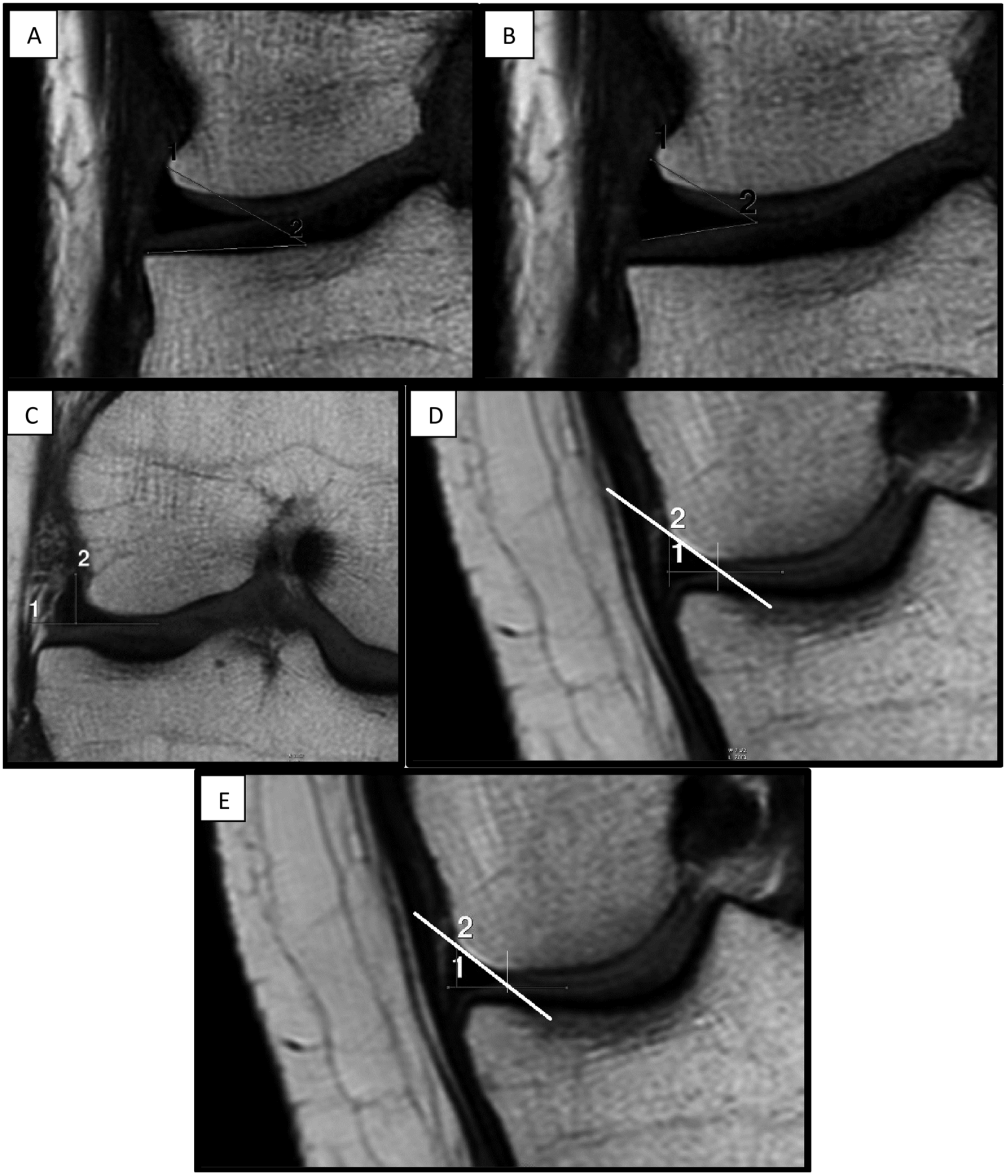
A-E. The central slice of a coronal MRI imaging of the knee focused on lateral compartment: A) meniscus-bone angle, B) meniscus-cartilage angle, C) meniscus-cartilage height, D) slope A, E) slope angle.
